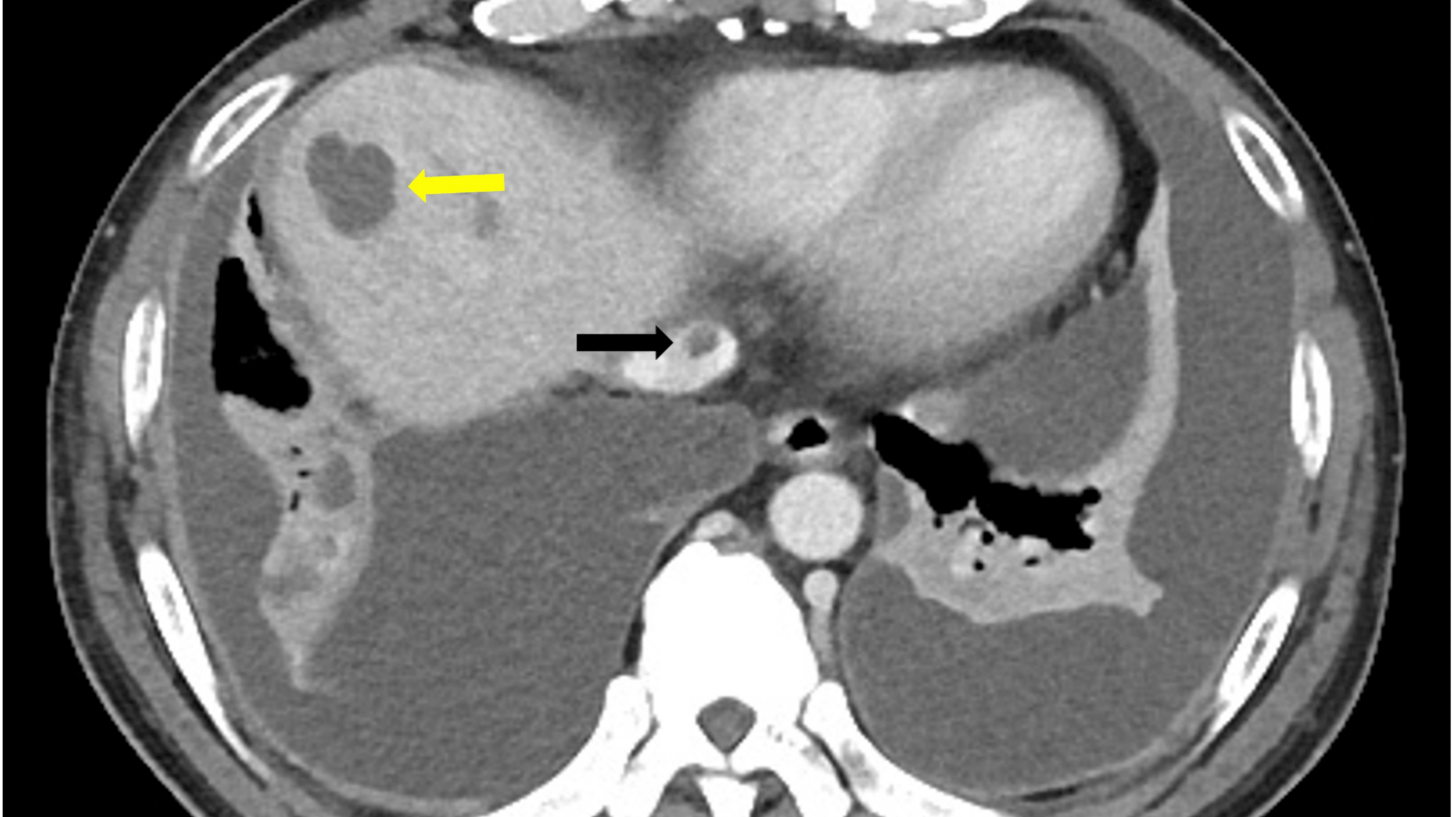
Contrast-enhanced abdominal computed tomography (CT) revealed liver abscess (yellow arrow) and inferior vena cava thrombosis (black arrow).
